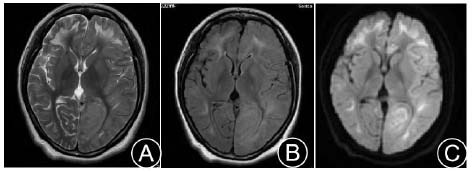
Cranial MRI: Symmetrical large patchy high signals in the corticomedullary junction and centrum semiovale of both cerebral hemispheres on T2WI and FLAIR (A, B); DWI shows band-like high signals in the corticomedullary junction of both cerebral hemispheres (C).
